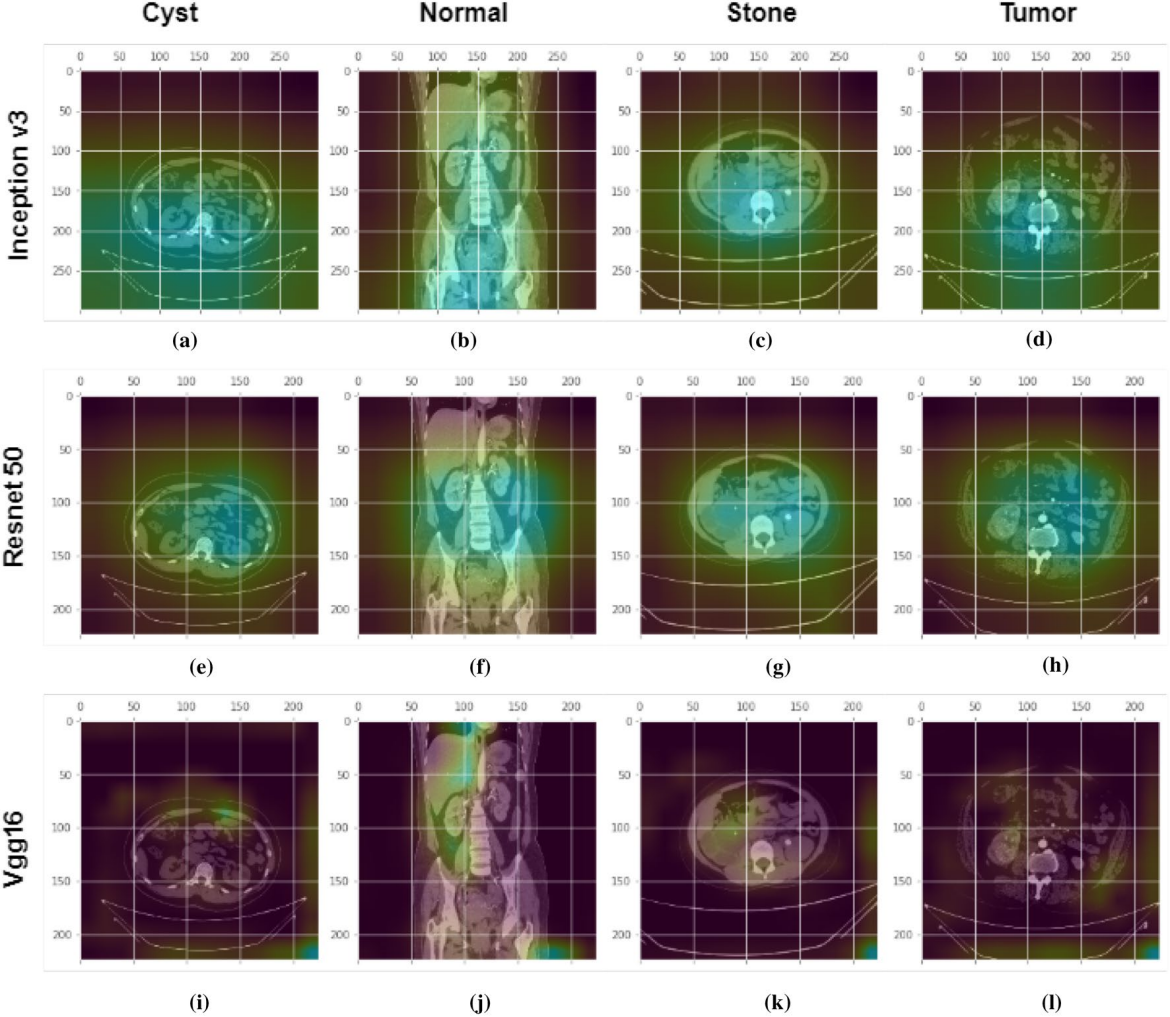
GradCam analysis of kidney Cyst, Normal, Stone and Tumor class photos at the final convolution layer in the Inception v3, Vgg16, and Resnet models. First row: shows the Gradcam images from inception v3 model for different classes. Second row: shows the Gradcam images from Resnet50 model for different classes. Third row: shows the Gradcam images from Vgg16 model for different classes. The GradCam activation mapping for the xray image is shown in the second row. The first, second, third, and fourth columns are for kidney cysts, normal, stone, and tumor classes respectively.
